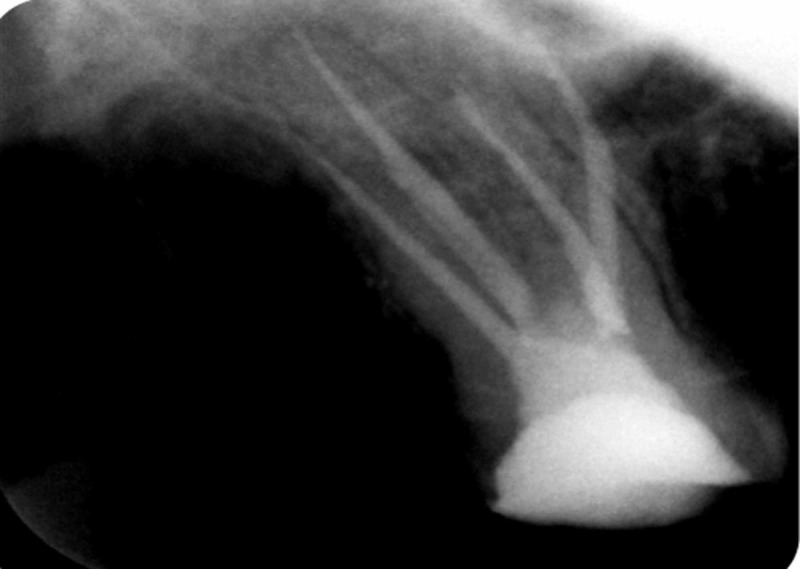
Obturation radiograph of #27
